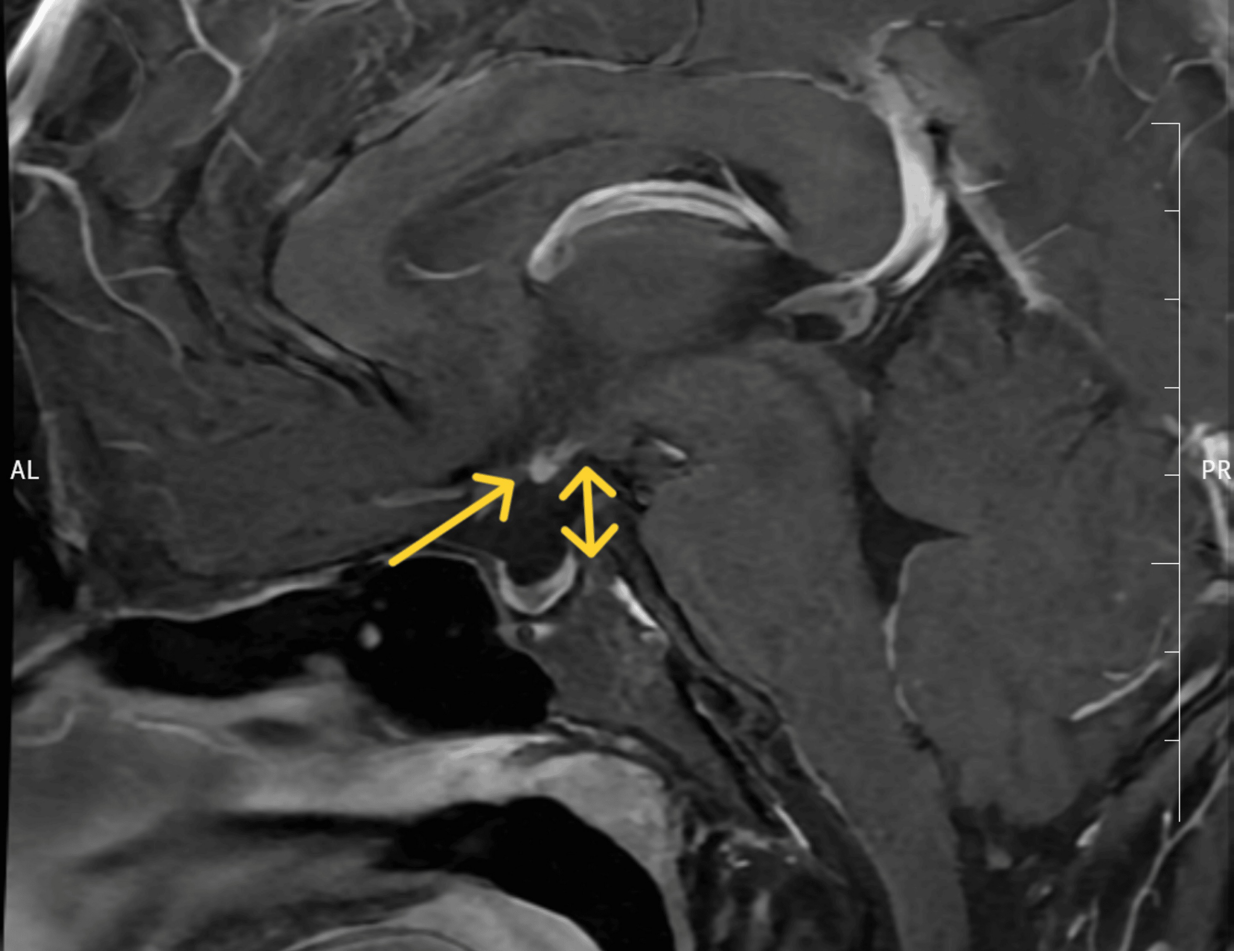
T1-weighted contrast enhanced MRI of sella turcica showing an ectopic posterior pituitary (yellow arrow) and absent pituitary infundibulum in Case 3
